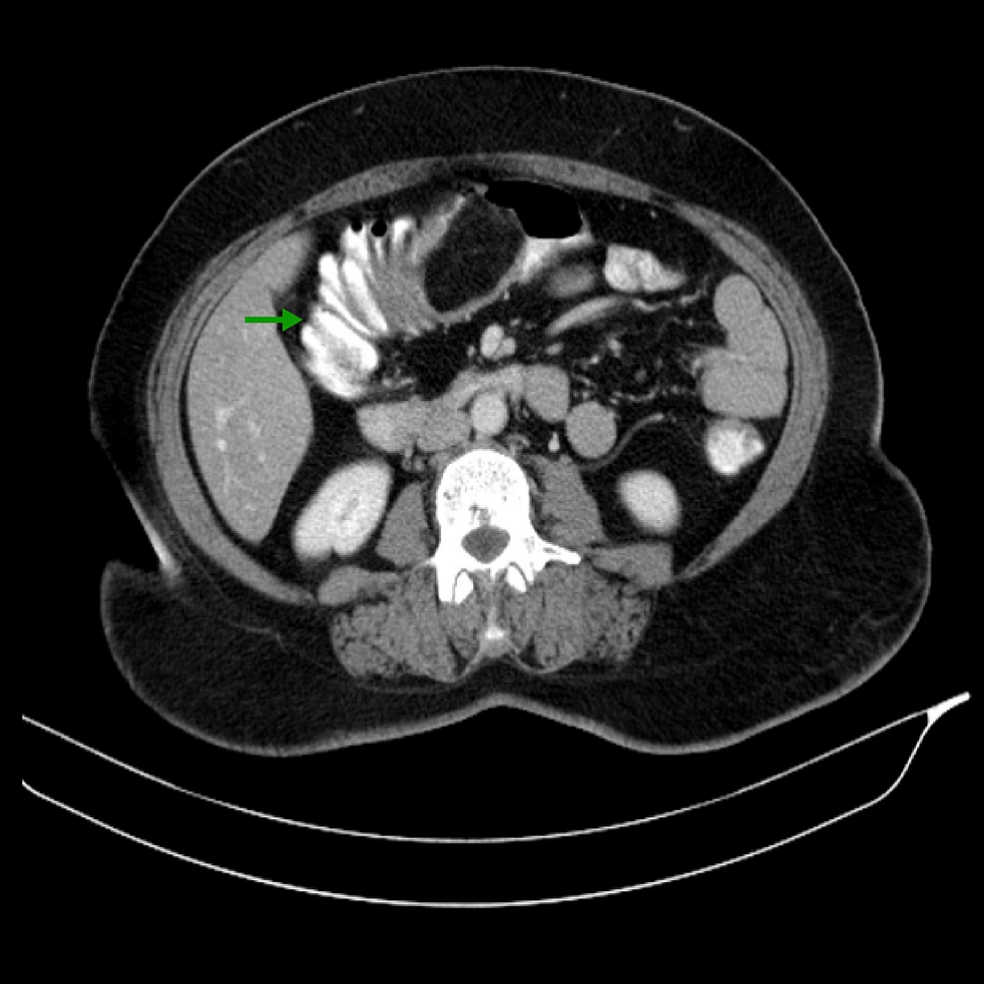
CT abdomen (axial section) The image shows fat density polypoidal lesion arising from ascending colon, prolapsing into the transverse colon (green arrow).
